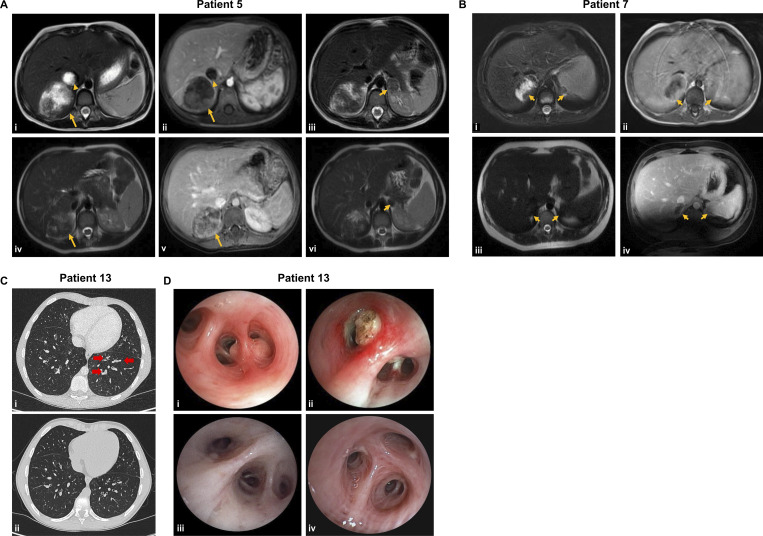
HCT halts progression and leads to regression of otherwise treatment-refractory EBV+ SMTs. (A) MRI images pre- (i, ii, iii; upper row) and 21 mo post-HCT (iv, v, vi; lower row) for P5: T2 weighted axial (i) and T1 weighted post-contrast axial (ii) images demonstrate two lesions in right adrenal gland (larger 62 mm) with T2 heterogenous signal and peripheral contrast enhancement (arrows and arrowhead), the smaller compressing the inferior vena cava (VCI). T2 weighted axial (iii) image shows lesion in left adrenal gland (arrow) with similar signal changes. After HCT, T2 weighted axial (iv) and T1 weighted post-contrast axial (v) images show a decrease in size of the larger right adrenal lesion (57 mm) and disappearance of the VCI-compressing lesion. T2 weighted axial image (vi) shows a similar decrease in size on the left adrenal lesion (size not reported). (B) MRI images pre- (i, ii; upper row) and 28 mo post-HCT (iii, iv; lower row) for P7: T2 weighted axial (i) and T1 weighted post-contrast axial (ii) images show bilateral adrenal lesions (right: 38 × 32 × 38 mm, left: size not reported) with T2 heterogenous signal and peripheral contrast enhancement (arrows). Post-HCT T2 weighted axial (iii) and T1 weighted post-contrast axial (iv) images show decrease in size of bilateral adrenal lesions (right: 25 × 17 mm; left: size not reported). (C) Axial CT images for P13 show multiple bronchial obstructions by intraluminal leiomyomas pre-HCT (i; red arrows) with complete resolution 10 mo after HCT (ii). (D) Bronchoscopy images for P13 show multiple intraluminal bronchial leiomyomas 2 mo pre-HCT (i), which relapsed following removal noted 1 mo pre-HCT (ii). Subsequent complete remission was seen at 11 mo (iii) and 24 mo (iv) after HCT. Images are representative and may not depict the same airway localization for pre- and post-HCT findings.
